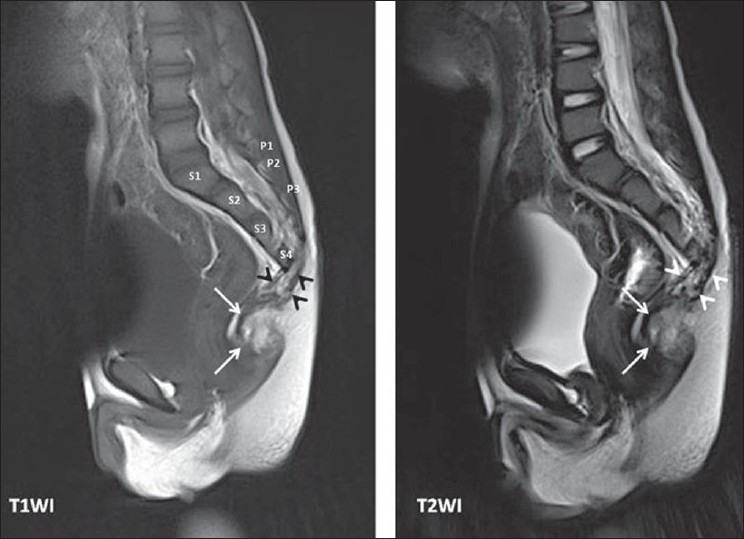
Sagittal T1-weighted and T2-weighted images show the mass (arrows) that extends into the spinal canal (arrow heads). S1, S2, S3 and S4 denote the body of the four sacral vertebrae and P1, P2 and P3 denote the posterior elements of the first to third sacral vertebrae in the T1 image
